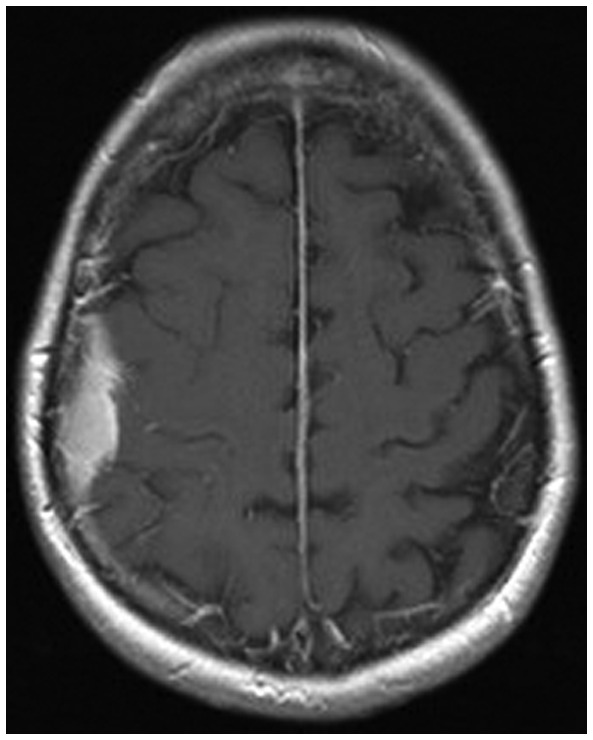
Preoperative MRI (T1 weighted image) showing an en plaque growing mass lesion with homogenous enhancement in the axial plane
